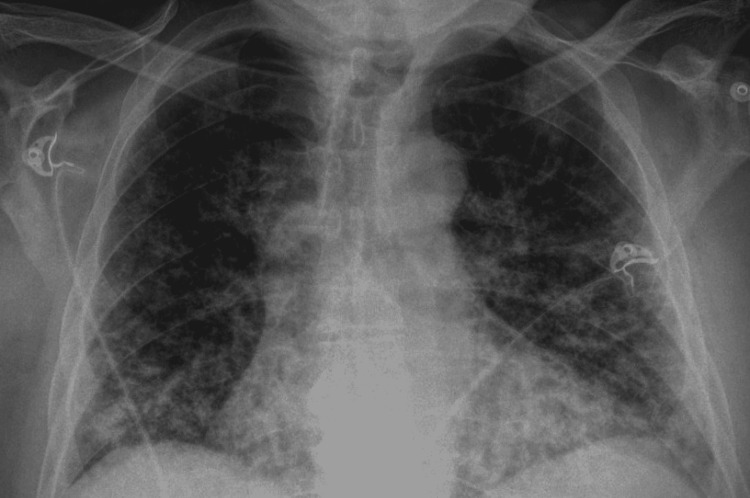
Initial Chest X-ray showing bilateral basilar lung infiltrates
